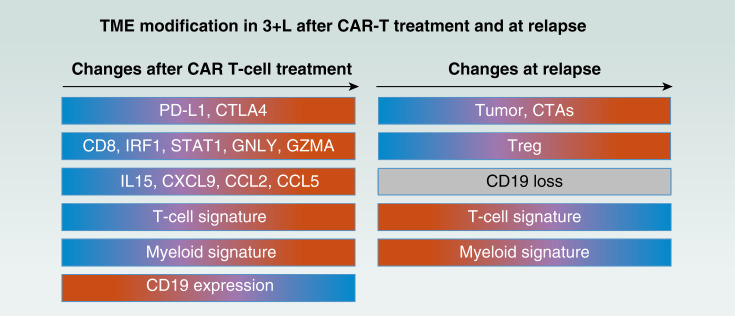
TME modification in 3L+ after CAR-T treatment and at relapse. Changes in immune cell profiles and signatures after CAR T-cell treatment are represented. Blue indicates low expression levels, red represents high expression levels, and gray reflects a complete loss of expression. A decrease in CD19 antigen expression and increase in immune cell biomarkers (PD-L1, CTLA4, CD8, IRF1, STAT1, GLNY, GZMA, IL15, CXCL9, CCL2, and CCL5), as well as in T-cell and myeloid signatures following CAR-T treatment, are shown. Changes at relapse are characterized by an increased expression of the cancer–testis antigen (CTA) expression, high levels of regulatory T cells (Tregs), and a reduction in myeloid and T-cell signatures. Complete loss of CD19 protein commonly occurs as a mechanism of relapse after CAR-T therapy. CCL2, chemokine ligand 2; CCL5, chemokine ligand 5; CD8, cluster of differentiation 8; CTLA4, cytotoxic T-lymphocyte–associated protein 4; CXCL9, C-X-C motif chemokine ligand 9; GNLY, granulysin; GZMA, granzyme A; IRF1, interferon regulatory factor 1; STAT1, signal transducer and activator of transcription 1.
